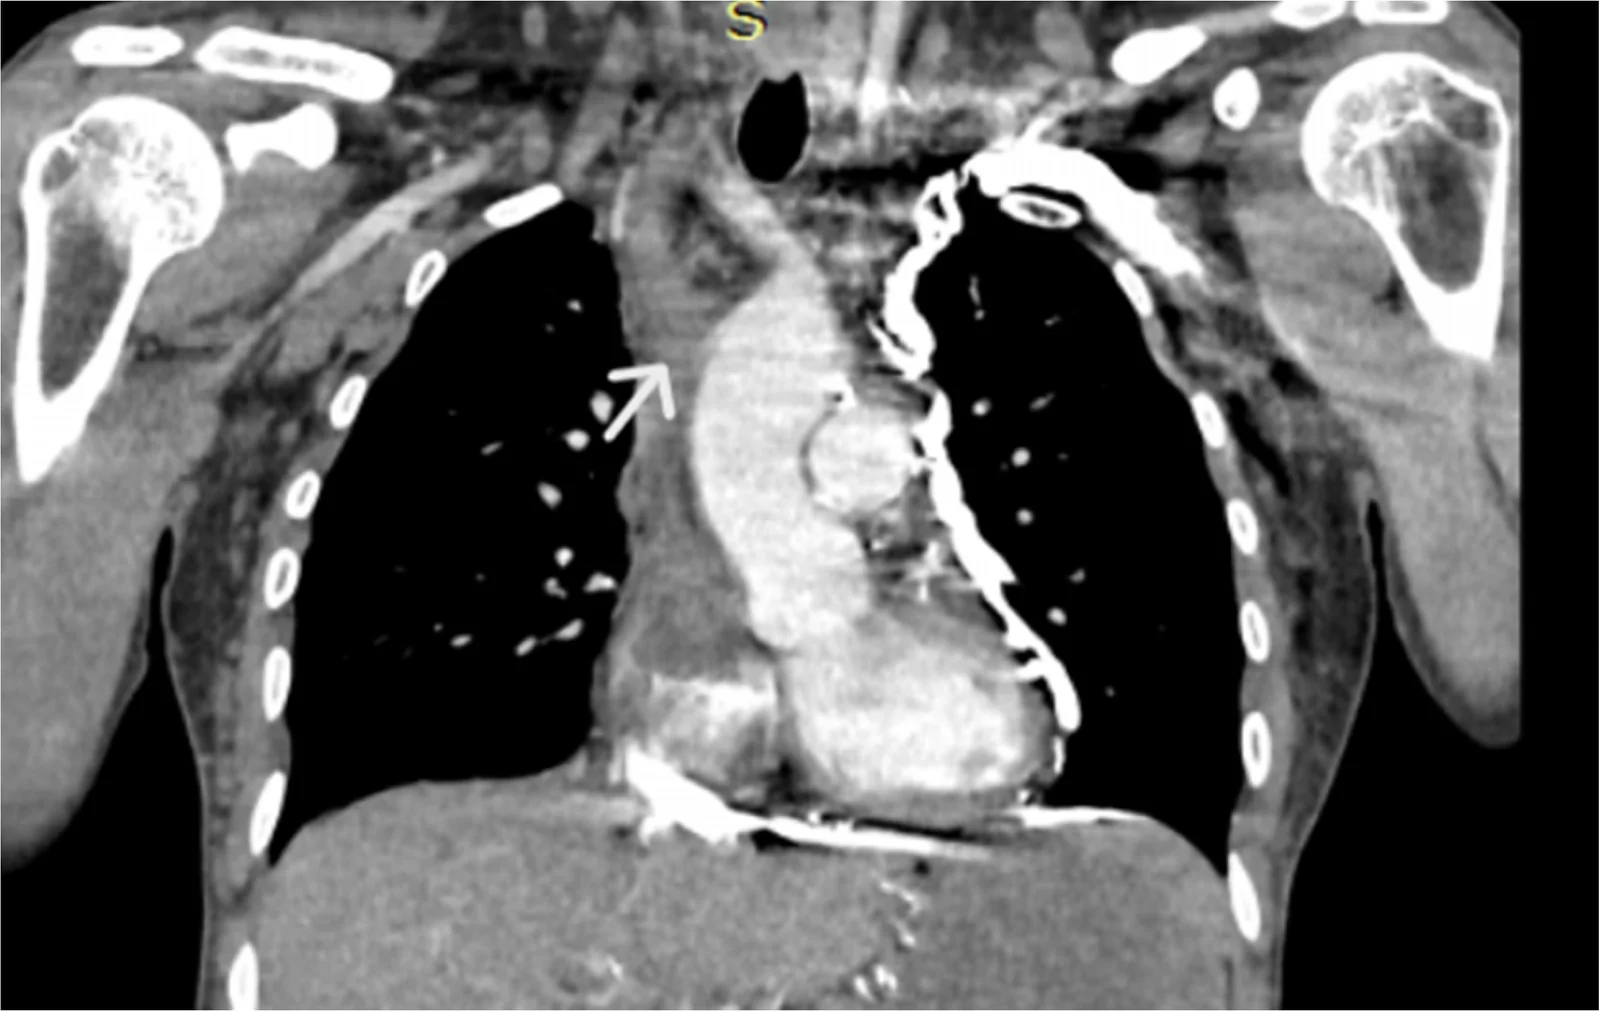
Coronal contrast-enhanced chest CT image showing a hypodense intraluminal filling defect (white arrow) within the superior vena cava (SVC), consistent with thrombotic occlusion. The superior vena cava appears partially obstructed, with extension of the thrombus along its course.
